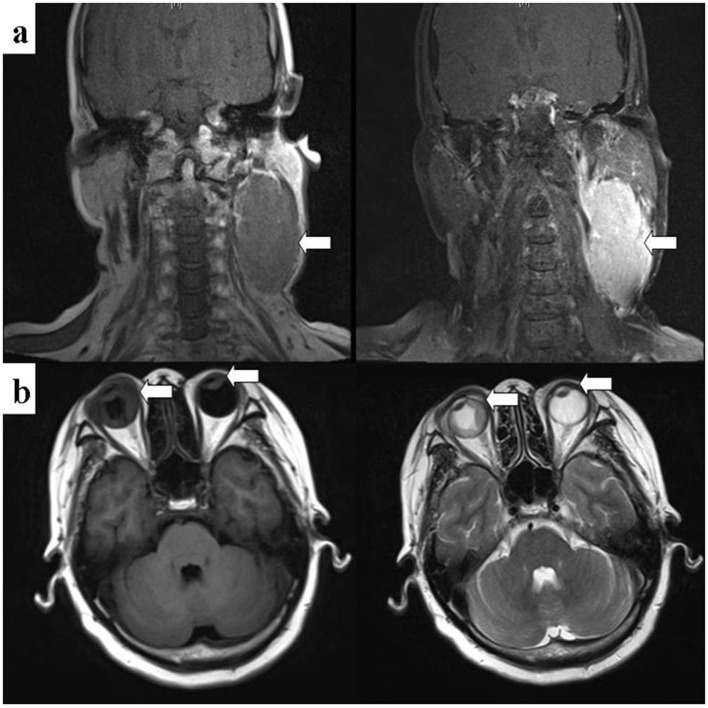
Magnetic resonance imaging (MRI) of the neck and eyeballs prior to therapy. (a) Subcutaneous mass (∼78.3×42.5 mm) in the left side of the neck. (b) The soft tissue located in the right eye socket, was enhanced markedly following the infusion of a contrast-enhancing agent. The adjacent muscles were also enhanced markedly. A 6.9×2.8 mm soft mass was observed in the left anterior chamber and was enhanced markedly following the infusion of a contrast-enhancing agent.
